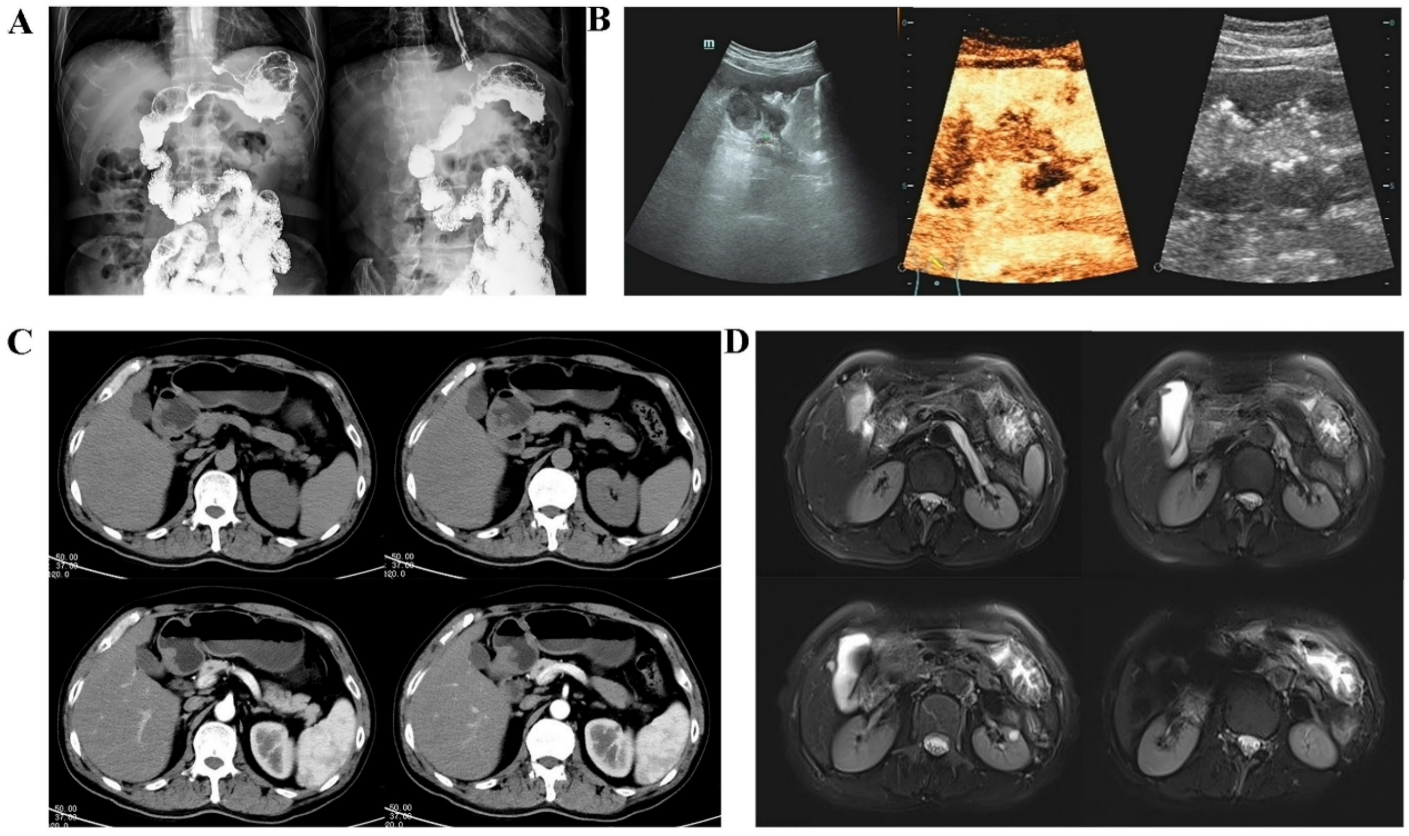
Typical images of imaging techniques of gastric cancer screening. (A): UGI: Localized rigidity of the gastric lumen, loss of normal mucosal morphology, and filling defects are observed. (B): Gastric ultrasonography: After oral administration of gastric window contrast agent, there are moderate echoes in the stomach, low echoes in the body of stomach, involving the body of stomach for one week, the range is about 116 × 41 mm, and local ulcers were found. Several hypoechoic lesions are found in the margin of the lesion, and the largest one is about 15 × 6 mm in size. (C): MDCT: The stomach is well filled, and the gastric wall of the gastric sinus is uneven and slightly thickened. After strengthening, it is slightly strengthened. (D): MRI: The mucosa of the greater curvature of the gastric body seemed to be thickened slightly and showed high signal intensity on DWI.
